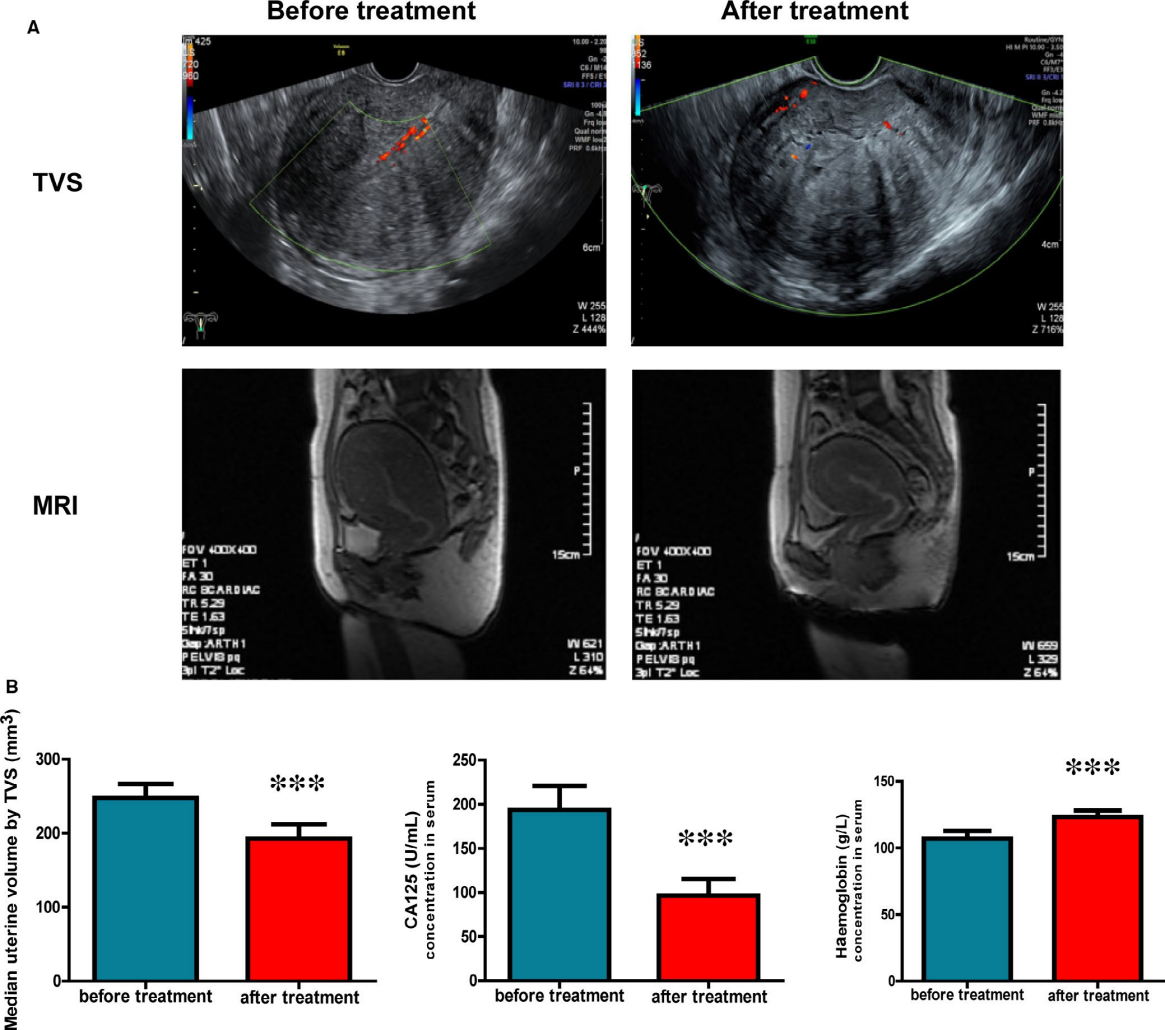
Mifepristone could be applied in the treatment of adenomyosis. A, Representative uterine images of TVS and MRI in the same adenomyosis patient before and after three‐month treatment with mifepristone. B, Median uterine volume was calculated in 20 adenomyosis patients before and after mifepristone treatment. Ultrasonography was applied to measuring the length of the long‐ and short‐axis of the sagittal section and the long‐axis of the cross section of the uterus. Uterine volume was assessed using the formula for an ovoid (length × width × depth × 0.52). The CA125 concentration and haemoglobin concentration in serum of adenomyosis patient were measured before and after three‐month treatment with mifepristone. MRI, magnetic resonance imaging; TVS, transvaginal ultrasound. Data were expressed as mean ± SEM. P* < .05, *P* < .01 and P* < .001
